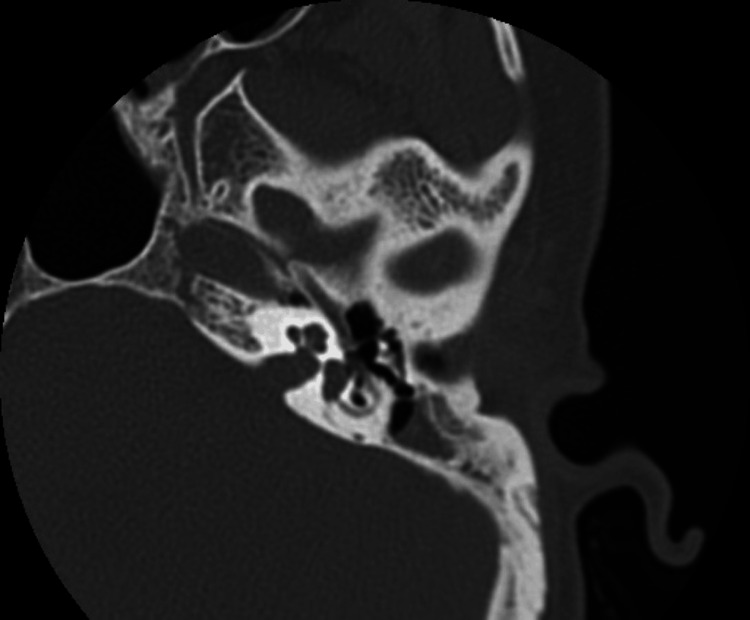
Sample error image of a misclassified SCOM CT scan image using the SVM model SCOM: suppurative chronic otitis media; CT: computed tomography; SVM: Support Vector Machine.
